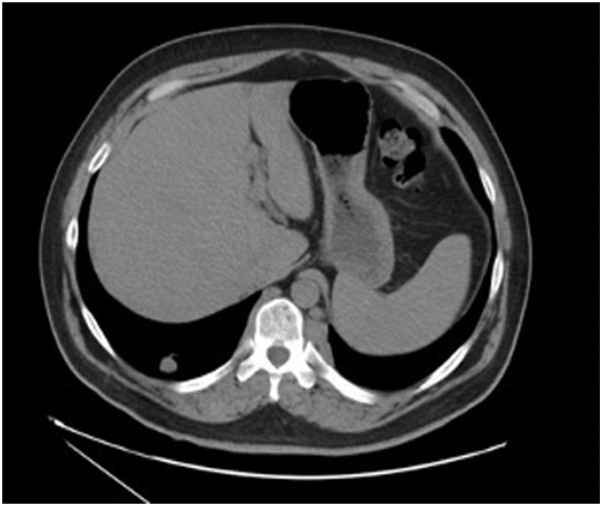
Computed tomography scan of right lower lobe nodule of the lung.
